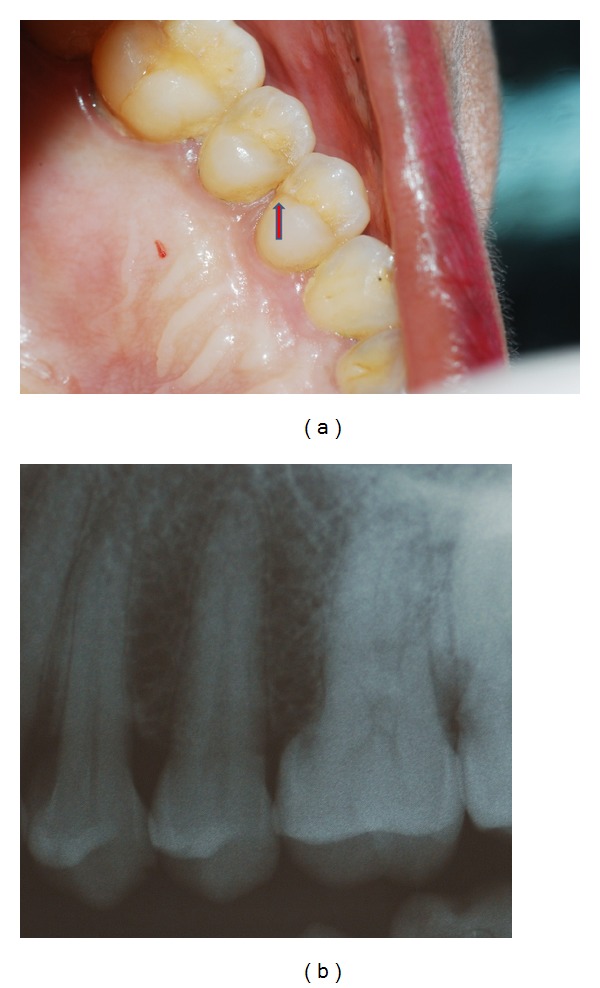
(a) Faint fracture line can be detected on distal marginal ridge extending onto proximal surface of 24. (b) Intraoral periapical radiograph of 24 reveals no significant findings.
